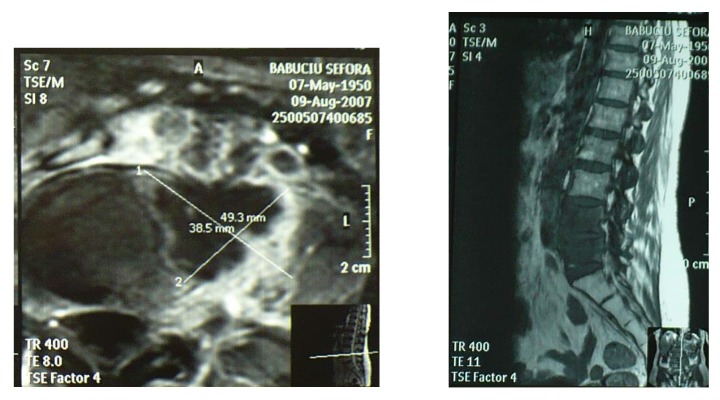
MRI images – invading retroperitoneal tumoral formation in vertebrae L4,L5
